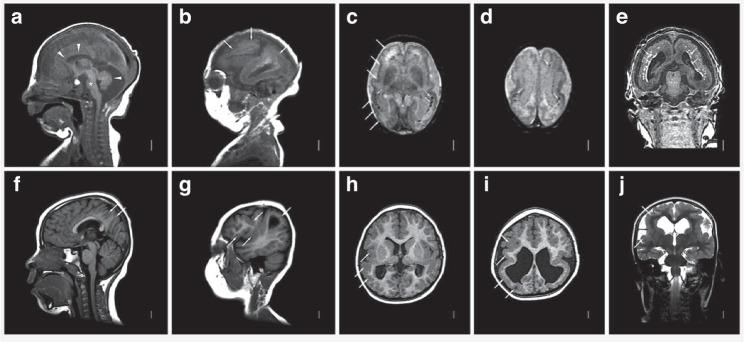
MRI scan findings in CACMV. This image demonstrates brain MRI abnormalities observed in cCMV. Images from a child with CACMV (panels a–e) demonstrate diffuse thin corpus callosum (upper arrowheads in a), small pons (asterisk in a), diffuse cerebellar hypoplasia (lower arrowhead in a), diffuse reduced number and complexity of gyri and irregular cortex consistent with PMG seen in all regions (arrows in b, long white arrows shown only on the right in c), and extensive periventricular and subcortical white matter injury and calcifications (short white arrow in c–e), and mild-moderate ventriculomegaly. Images from another patient with CACMV show normal corpus callosum and cerebellum with PMG over the parietal convexity and right perisylvian region (arrows in f, g), bilateral perisylvian predominant PMG (long white arrow shown only on the right in (h–j), and moderate posterior ventriculomegaly. The images include T1-weighted sagittal (a, b, f, g), T2-weighted non-turbo axial (c, d), 3D-MPRAGE coronal (e) and axial (h, i) and T2-weighted coronal (j) sequences.
